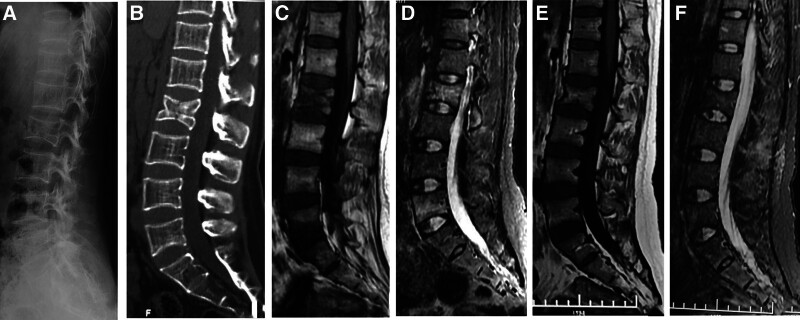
(A) The lateral X-ray film of the patient shows the compression change in L2 vertebral body on admission. (B) CT images reveals compression change and abnormal density in the L2 vertebral body on admission. (C) T1-weighted MRI shows abnormal low signal changes in the L2 vertebral body, lower edge of L5 vertebral body, and upper edge of S1 vertebral body on admission. (D) T2-weighted fat-suppressed MRI shows abnormal high signal changes in the L2 vertebral body, lower edge of L5 vertebral body, and upper edge of S1 vertebral body on admission. (E) After 3 months of conservative treatment, T1-weighted MRI shows that the abnormal signal in the affected vertebral body disappeared; (F) after 3 months of conservative treatment, T2-weighted fat-suppressed MRI shows that the abnormal signal in the affected vertebral body has disappeared. CT = Computer Tomography, MRI = magnetic resonance imaging.
